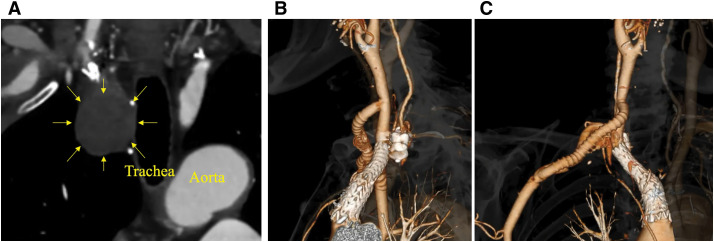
Fig. 3 Postoperative follow-up imaging. (A) Contrast-enhanced CT (coronal view, 1 month) demonstrating exclusion of the aneurysm. (B) Volume rendering reconstruction in right anterior oblique projection at 1 month showing no endoleak. (C) Volume rendering reconstruction in left anterior oblique projection at 1 month, confirming a bypass patency. CT: computed tomography
